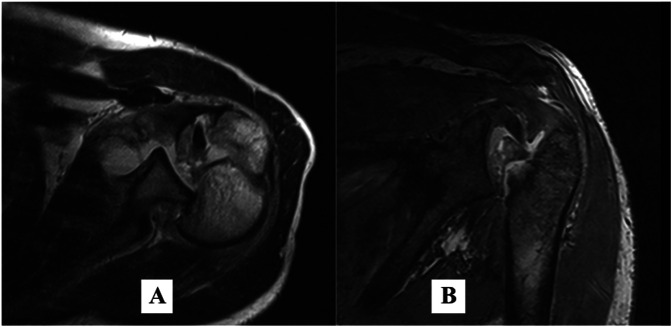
T2-axial (A) and T1-coronal (B) MRI of the left shoulder joint showing a displaced posterior fracture-dislocation.
